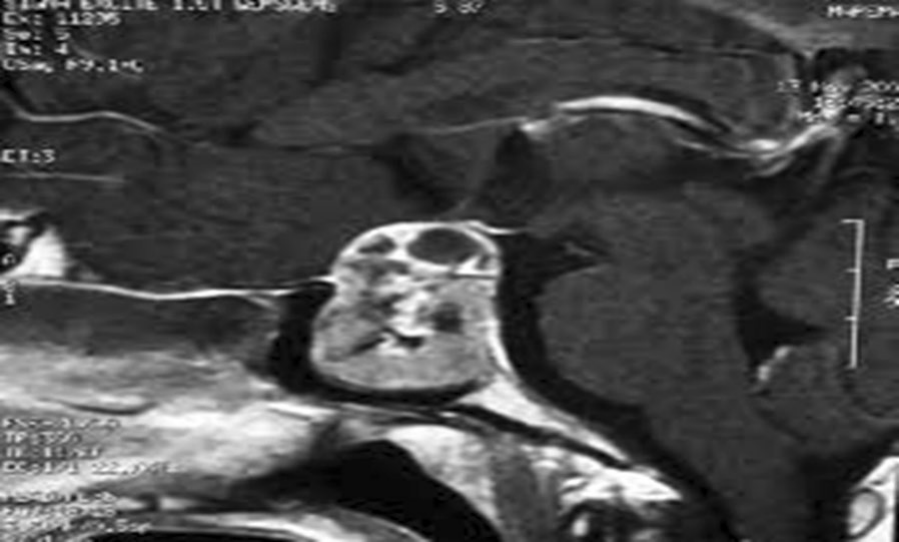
Enhanced sagittal T1 MR image of the patient. Sporadically hypointense areas indicative of calcification are detectable
